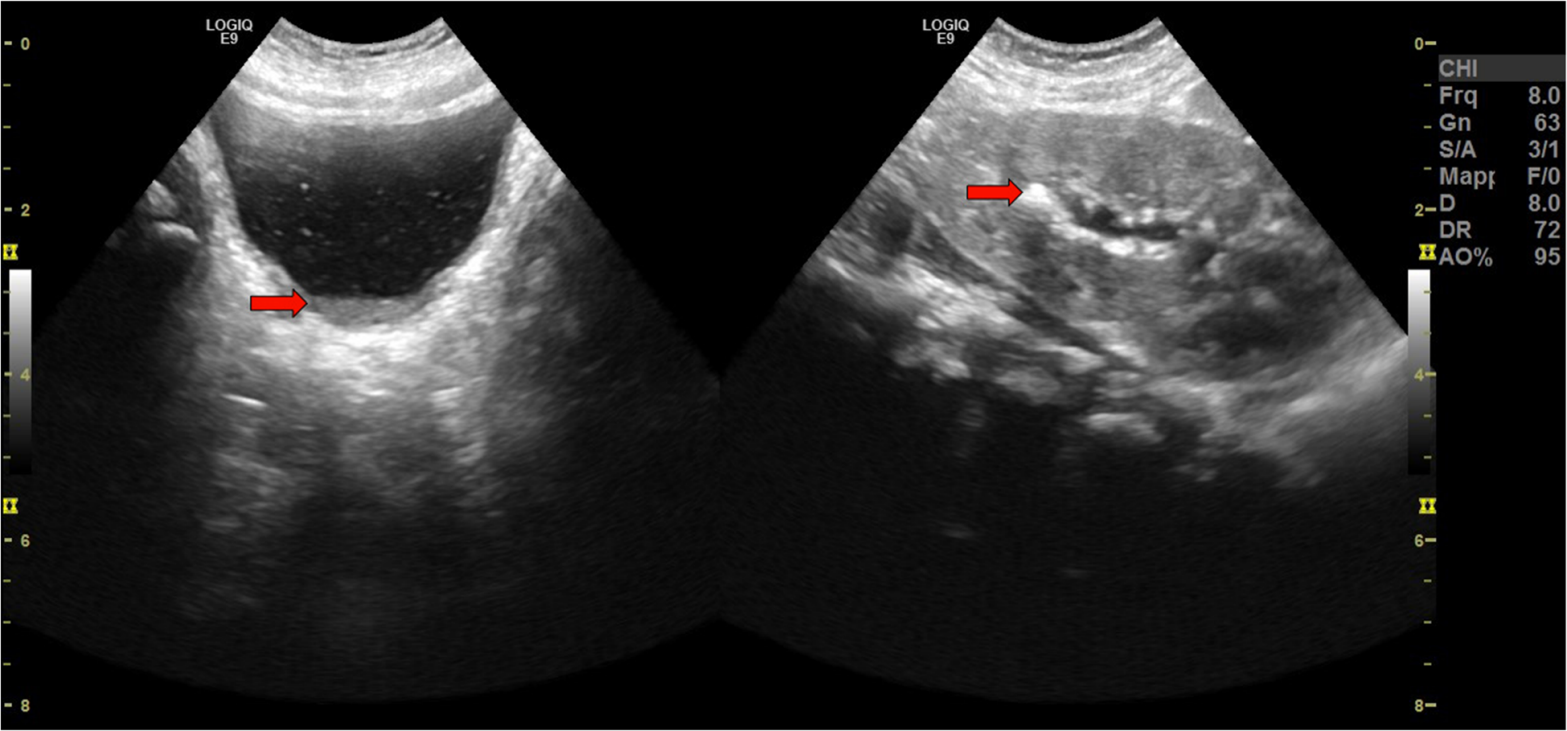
First patient’s renal and urinary tract ultrasound: small hyperechoic formations, compatible with calcium oxalate crystals, in the left kidney (the arrow indicates the largest, with maximum diameter of about 3 mm) and bladder (the arrow indicates their sediment)
